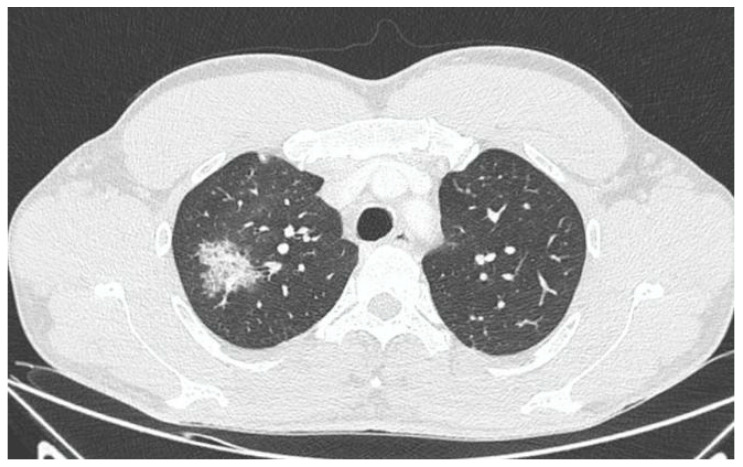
CT scan of the chest showing right lung upper lobe interstitial changes during first hospitalization.
